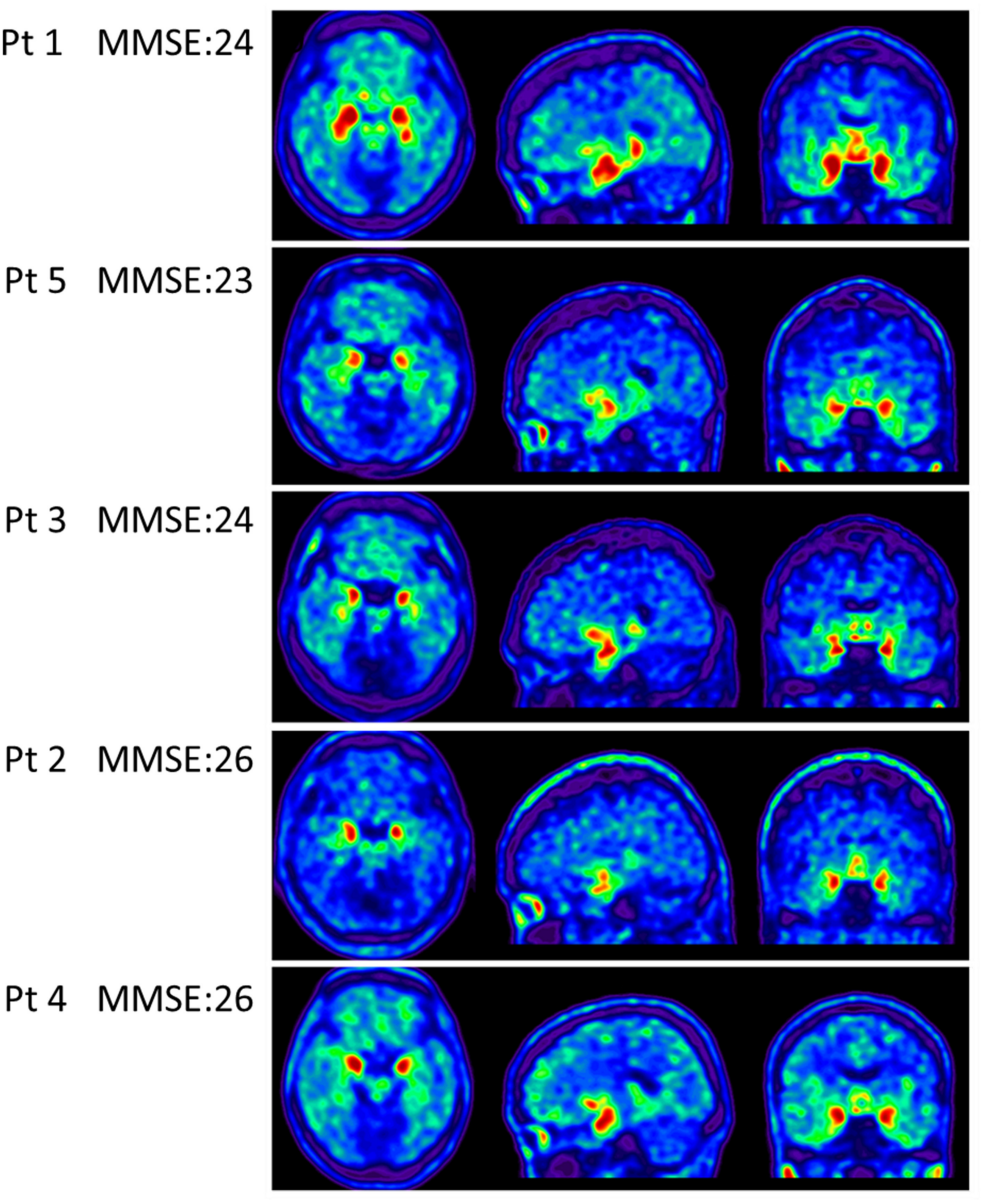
Tau PET scan with THK-5351 in the five patients with fMCI showing an increased SUVR of THK-5351 particularly extending to the lateral temporal area.
